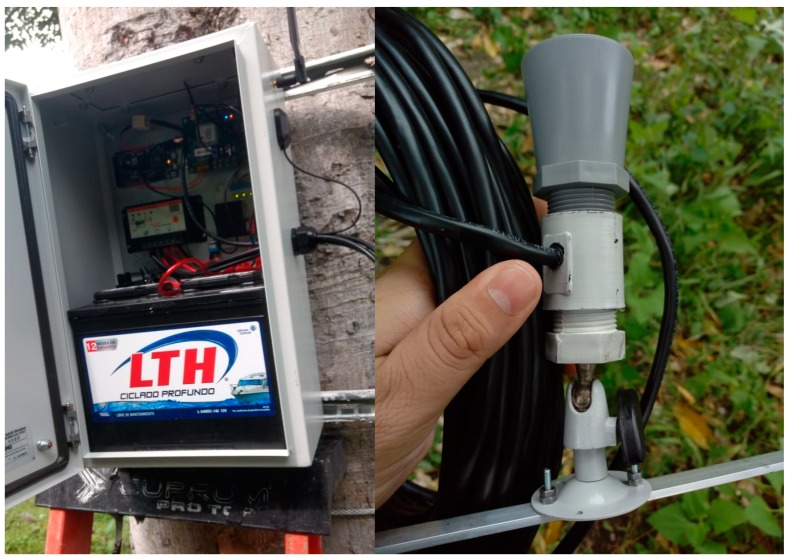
Electronic boards and ultrasonic sensor.
